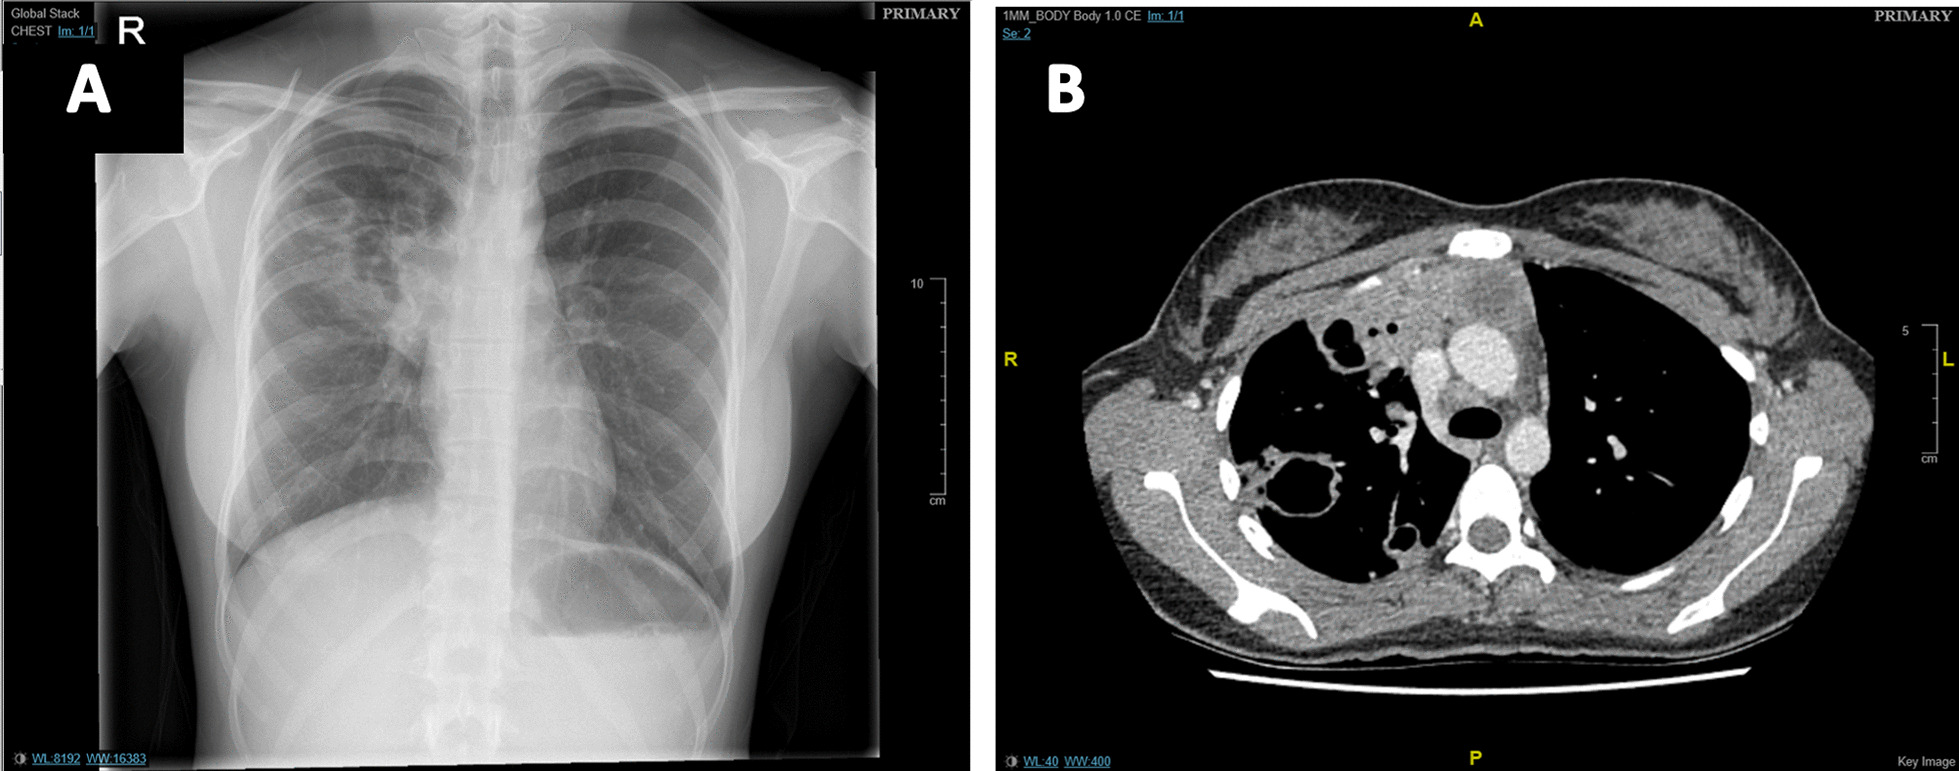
Imaging demonstrating cavitating lung lesions. A Chest X-ray demonstrating cavitating lesions in the right upper lobe. B Contrast-enhanced Chest CT scan showing cavitating lesions in the right lung with multiple nodular lesions bilaterally, along with an anterior mediastinal mass that is continuous with the posterior sternum
